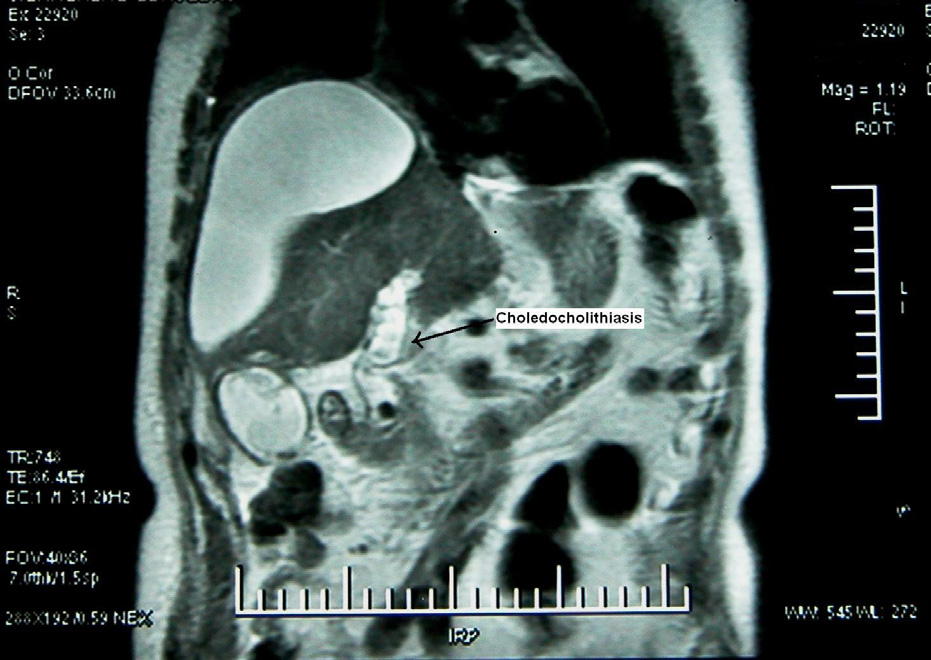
Abdominal MRI showing CBD stones.
